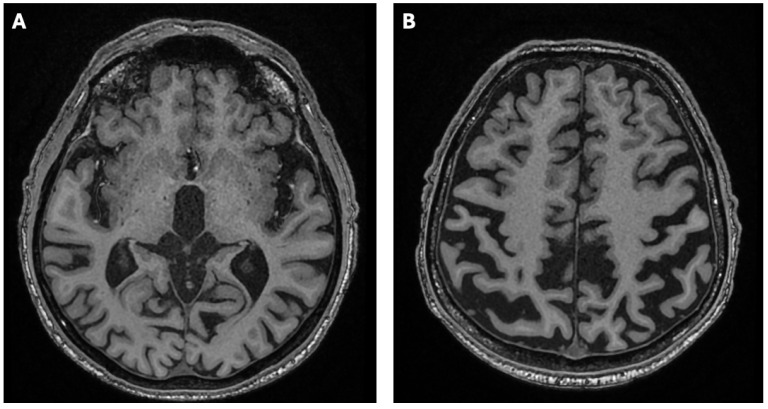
Brain MRI of the patient showing dilatation of the third ventricle on the T1-weighted sequence (A) and cortical atrophy (B).
